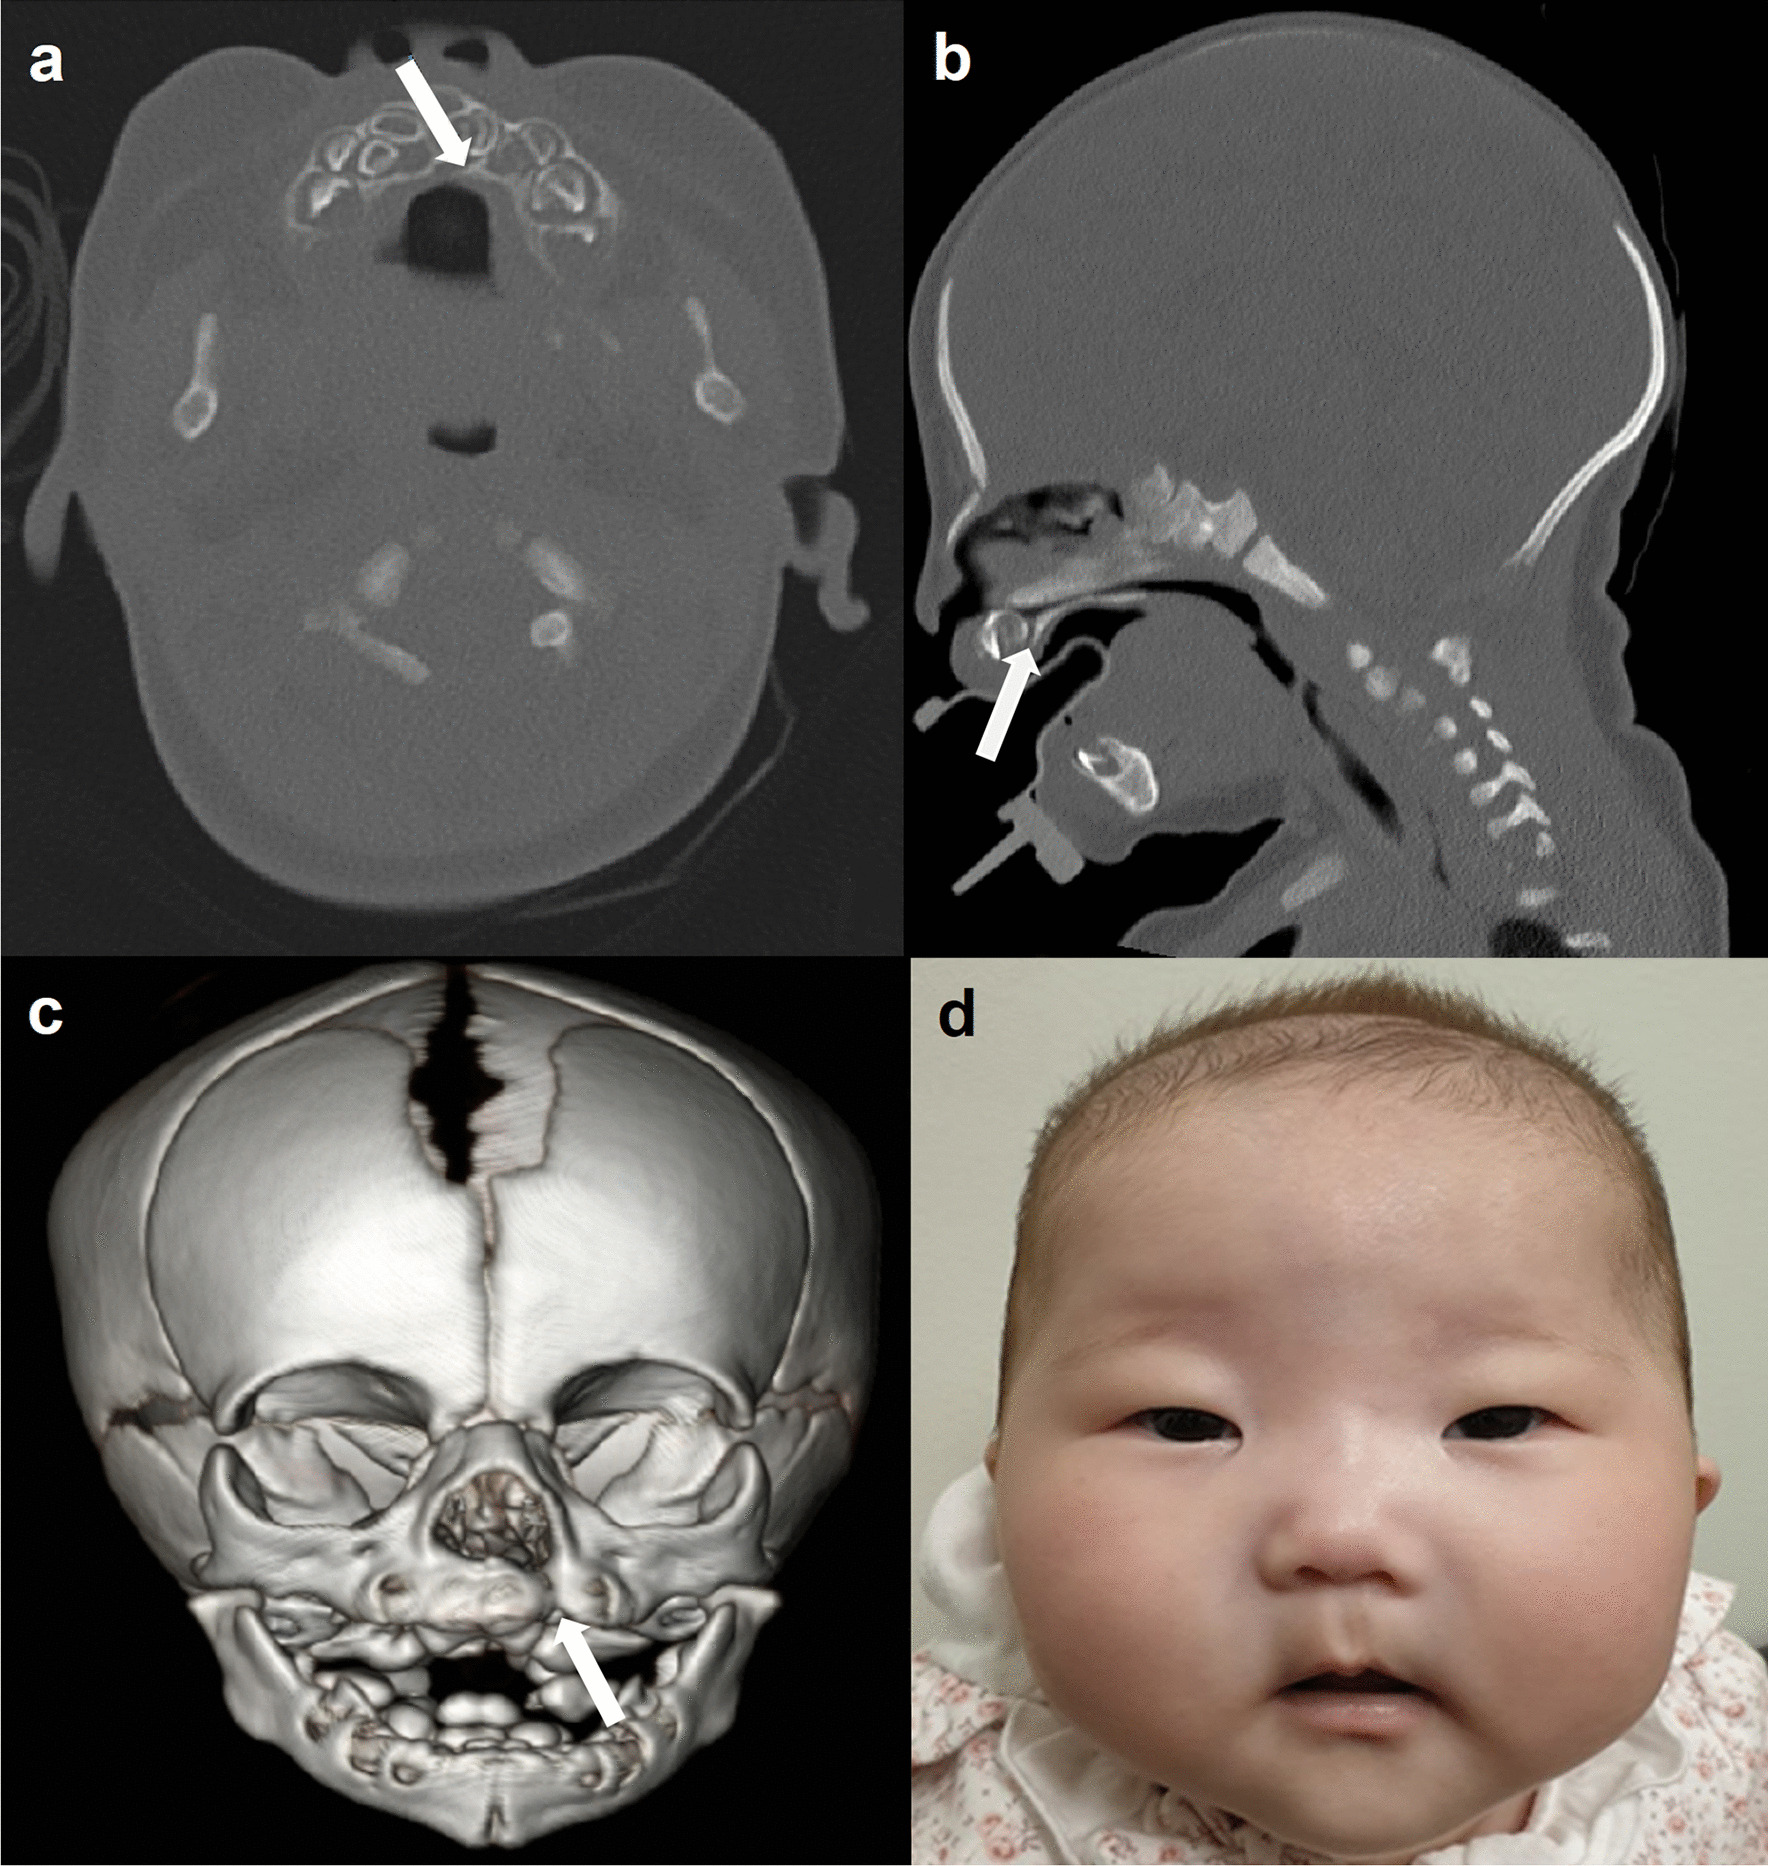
Facial computed tomography (CT) and photograph of the patient. a Axial, b coronal, and c three-dimensional reconstruction non-contrast CT images at three-weeks-of-age show unilateral bony defect (arrow) of the maxilla hard palate and mixed dentition. d Photograph of the patient
